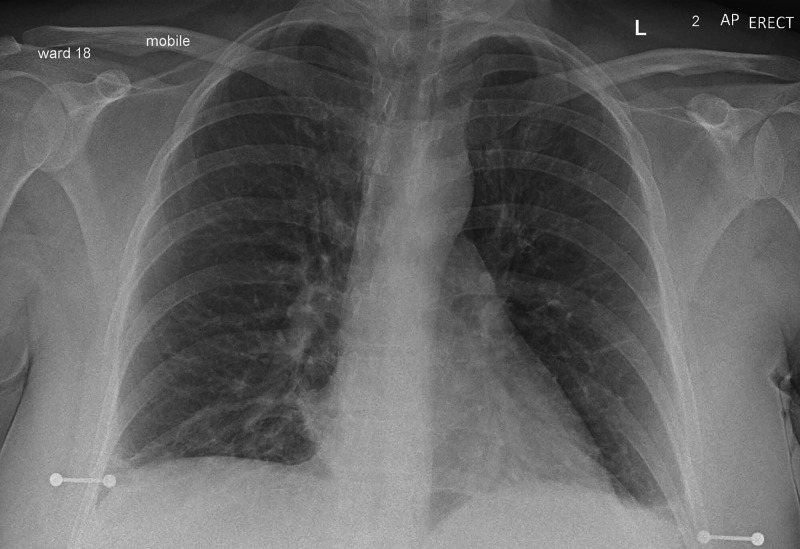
Normal Chest X-ray 26 hours post admission (24/09/2020)
